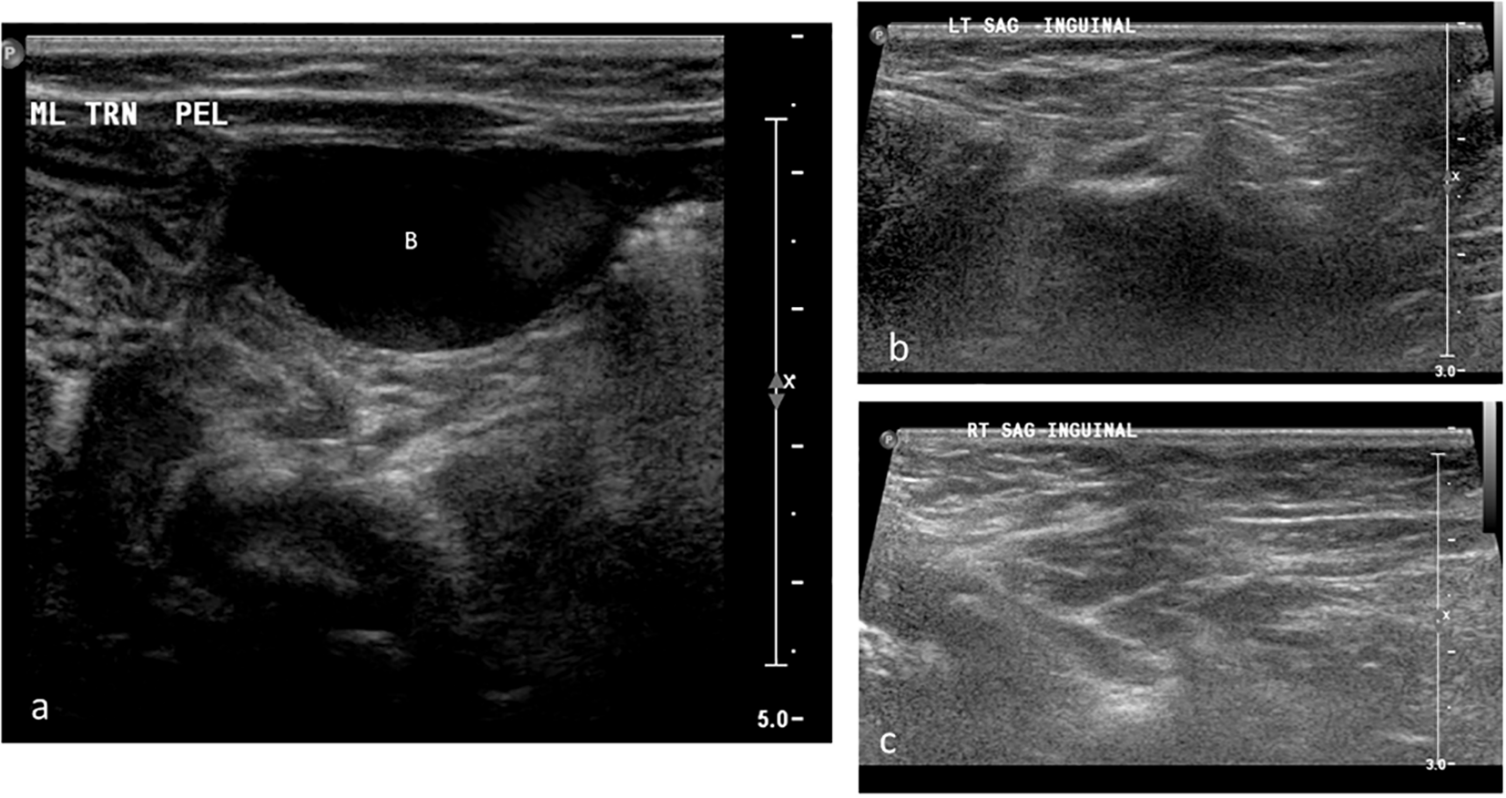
Grayscale ultrasound images of the pelvis and bilateral inguinal canals in Patient 1. (a) Transverse pelvic scan showing the urinary bladder (B); no uterus or gonadal structures are visualized posterior to the bladder. (b) Longitudinal scan of the left inguinal canal demonstrating absence of gonadal tissue (arrow). (c) Longitudinal scan of the right inguinal canal also showing no visible gonadal tissue.
